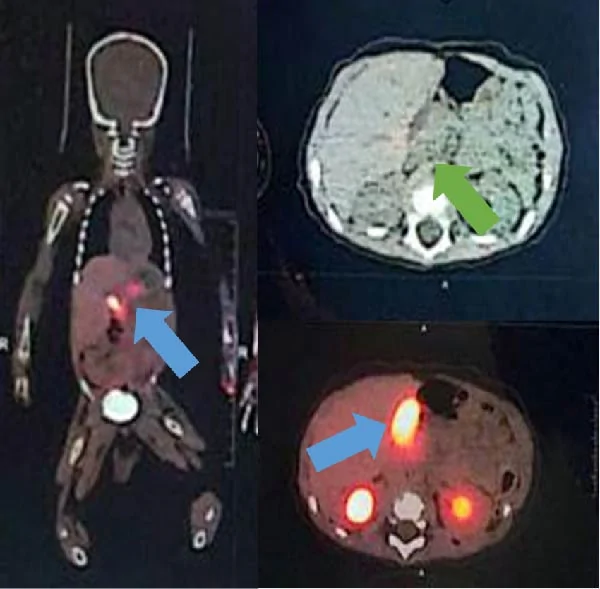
^18^F‐DOPA PET/CT demonstrating diffuse pancreatic uptake consistent with diffuse congenital hyperinsulinism.
